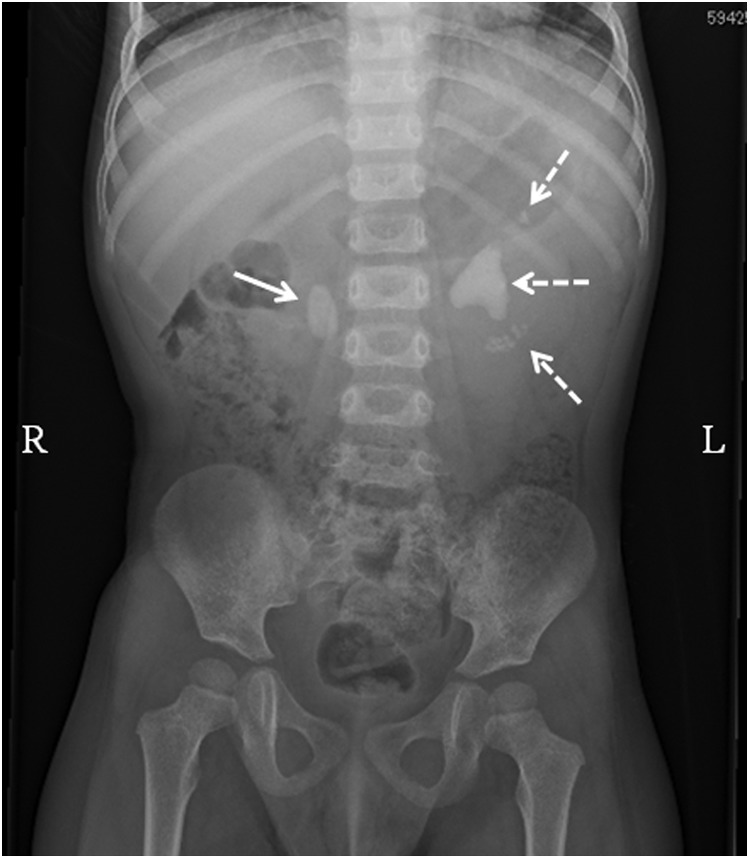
KUB radiograph in our 2-year-old patient. The *arrow* indicates the stone in the pyeloureteral junction of the right kidney. The *dotted arrow* indicates the large kidney stone with multiple stones in the upper and lower poles. KUB, kidney, ureter, and bladder.
